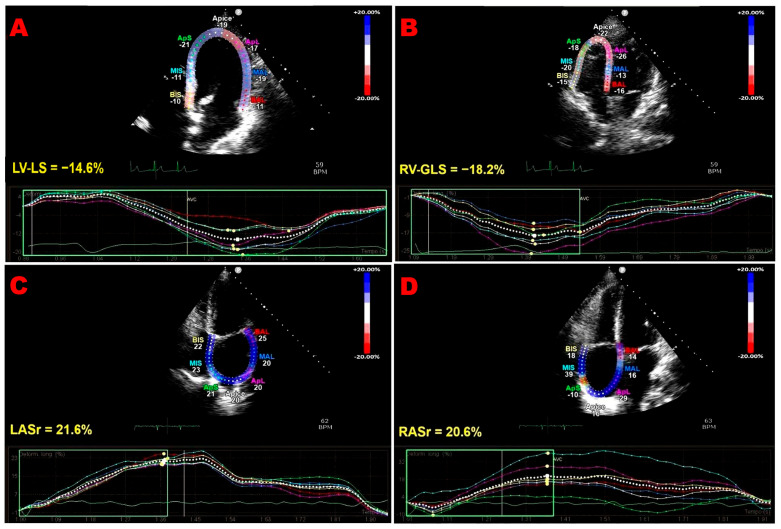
Representative examples of biventricular and biatrial myocardial strain parameters assessed by speckle-tracking echocardiography from the apical four-chamber (4C) view in an ICI-treated patient enrolled in the present study who developed ICI-related cardiac dysfunction. (A) LV-LS, left ventricular longitudinal strain. (B) RV-GLS, right ventricular global longitudinal strain. (C) LASr, left atrial reservoir strain. (D) RASr, right atrial reservoir strain. For each cardiac chamber, color-coded segmental strain maps are provided together with the corresponding strain curves. ICI, immune checkpoint inhibitors.
